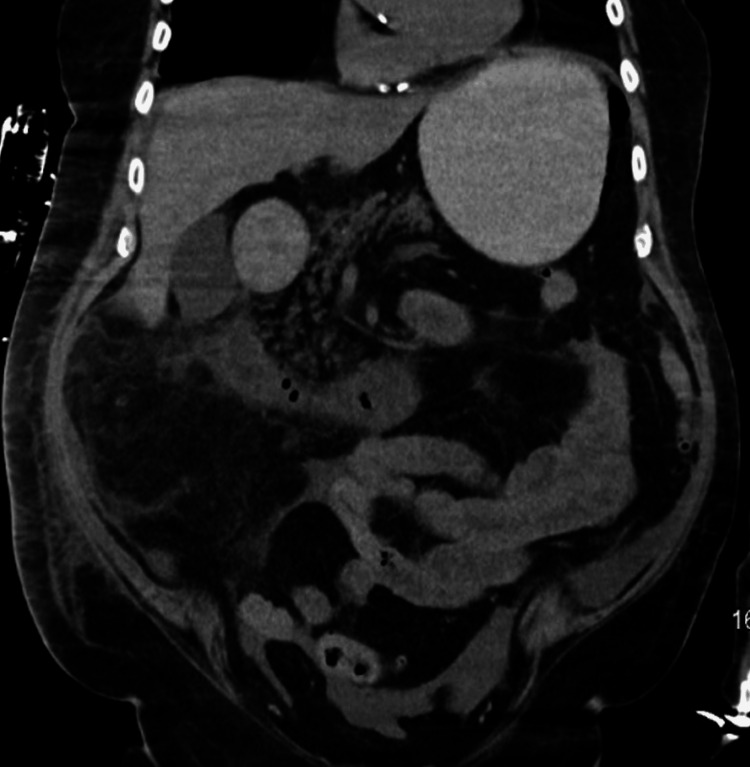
Abdominal CT-AP. CT-AP without contrast, showing free air and fluid surrounding the transverse colon, due to a possible perforated sigmoid diverticulitis vs post-surgical air and fluid. AP: anteroposterior view, CT: computed tomography.
